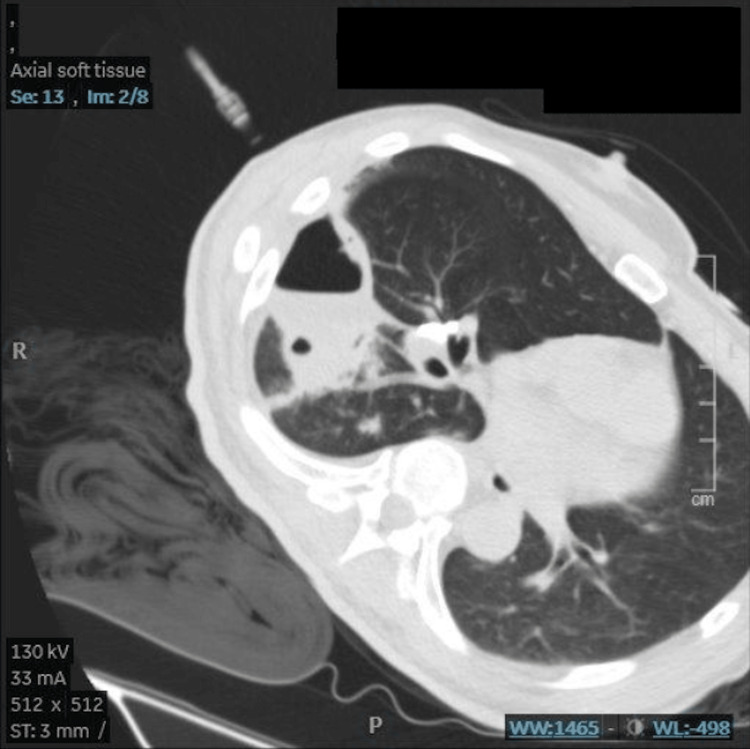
CT scan of the abscess post-drainage
